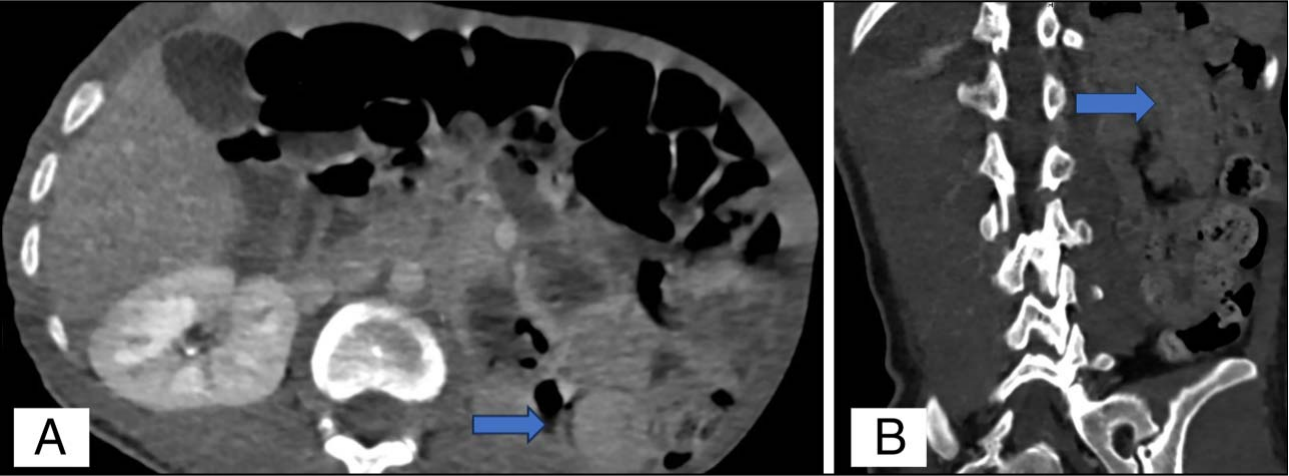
Abdominal and pelvic computed tomography axial (A) and coronal (B) sections demonstrating a circular focus of small bowel (jejunum, 5.5 cm) abutting the posterior abdominal wall, with a “target sign” appearance (blue arrow) within the posterior left upper quadrant suggestive of intussusception.
